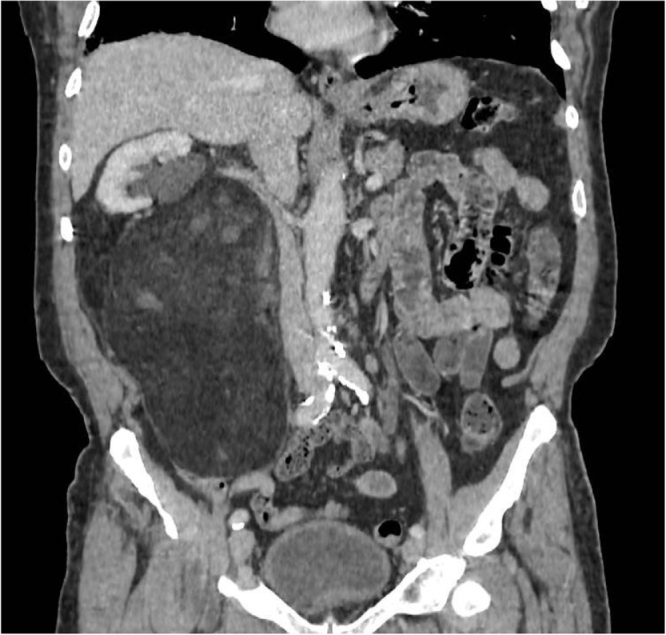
Preoperative CT scan of right liposarcoma.
